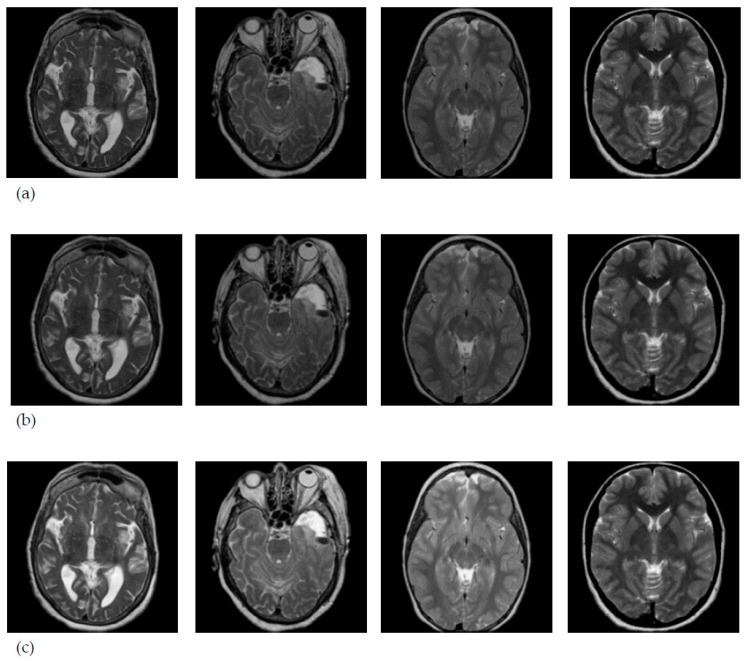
MRI brain images from the collected dataset (a) Original MRI images; (b) enhanced by the Gaussian filter with kernel of (3 × 3); (c) normalized MRI.
